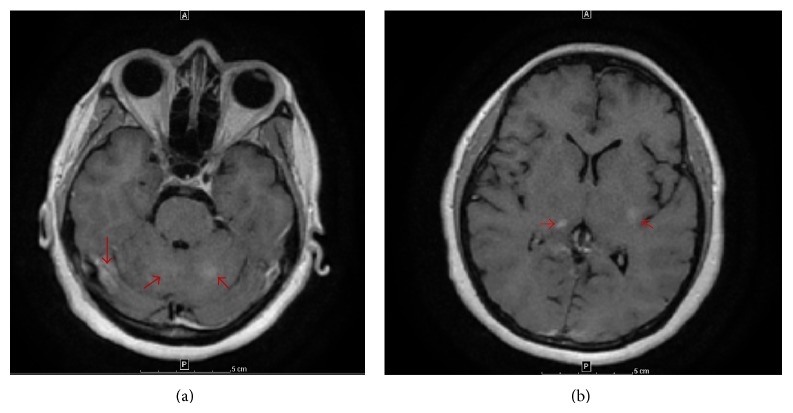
MRI brain: axial T1 images with contrast (a and b) demonstrating nodular enhancement (red arrows) in multiple areas of the brain.
